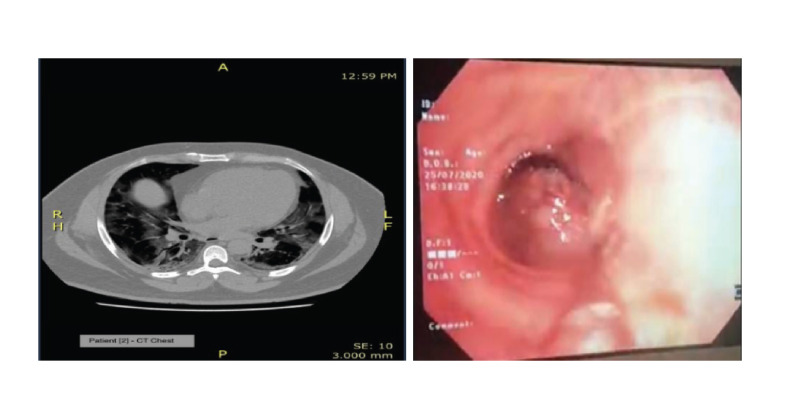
Computed tomography (A) scan for patient 2 and fibrinous plugs in subsegmental bronchi.
